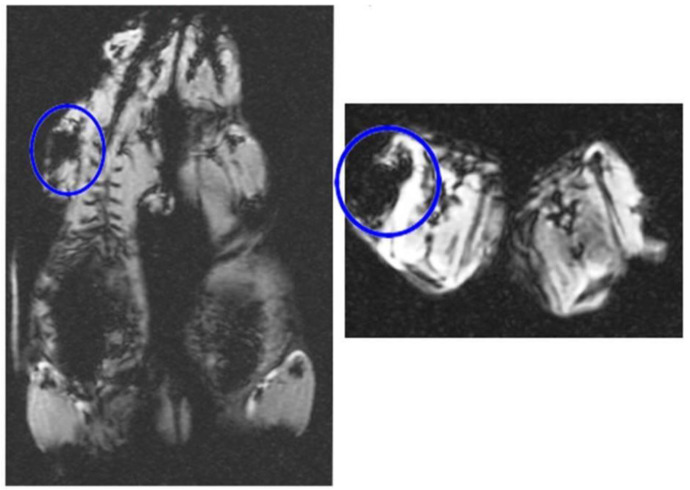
The T2* weighted images of in vivo samples showing the hypo-intense signal of 180 µg/mL of iron oxide labeled DPSCs transfected with 1 µL/mL lipofectamine that confirms presence of iron oxide in the labeled cells in comparison to in vivo samples of non-labeled cells showing lack of this MRI appearance (Left and Right).
